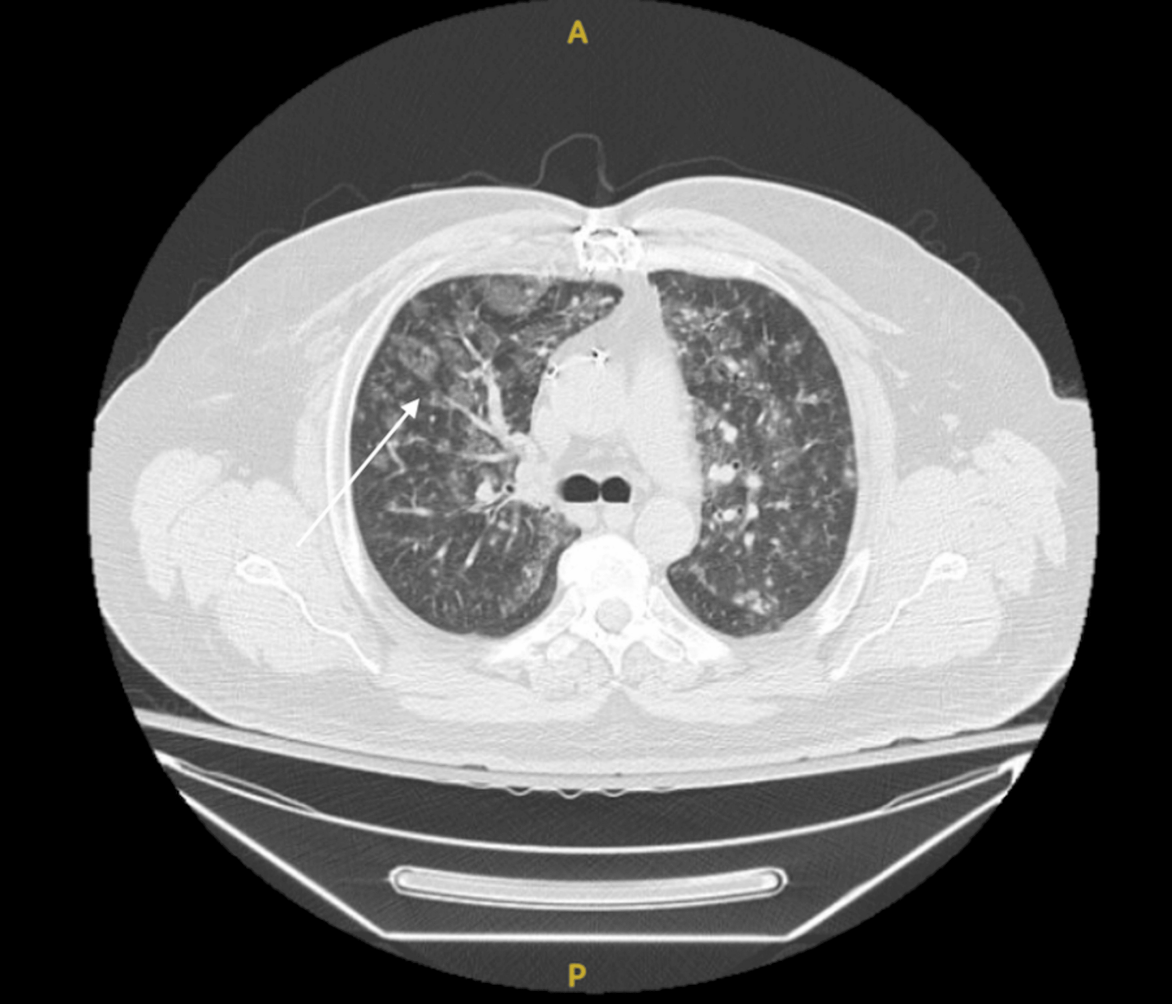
CT scan of the chest demonstrating ground-glass opacities CT: computed tomography
